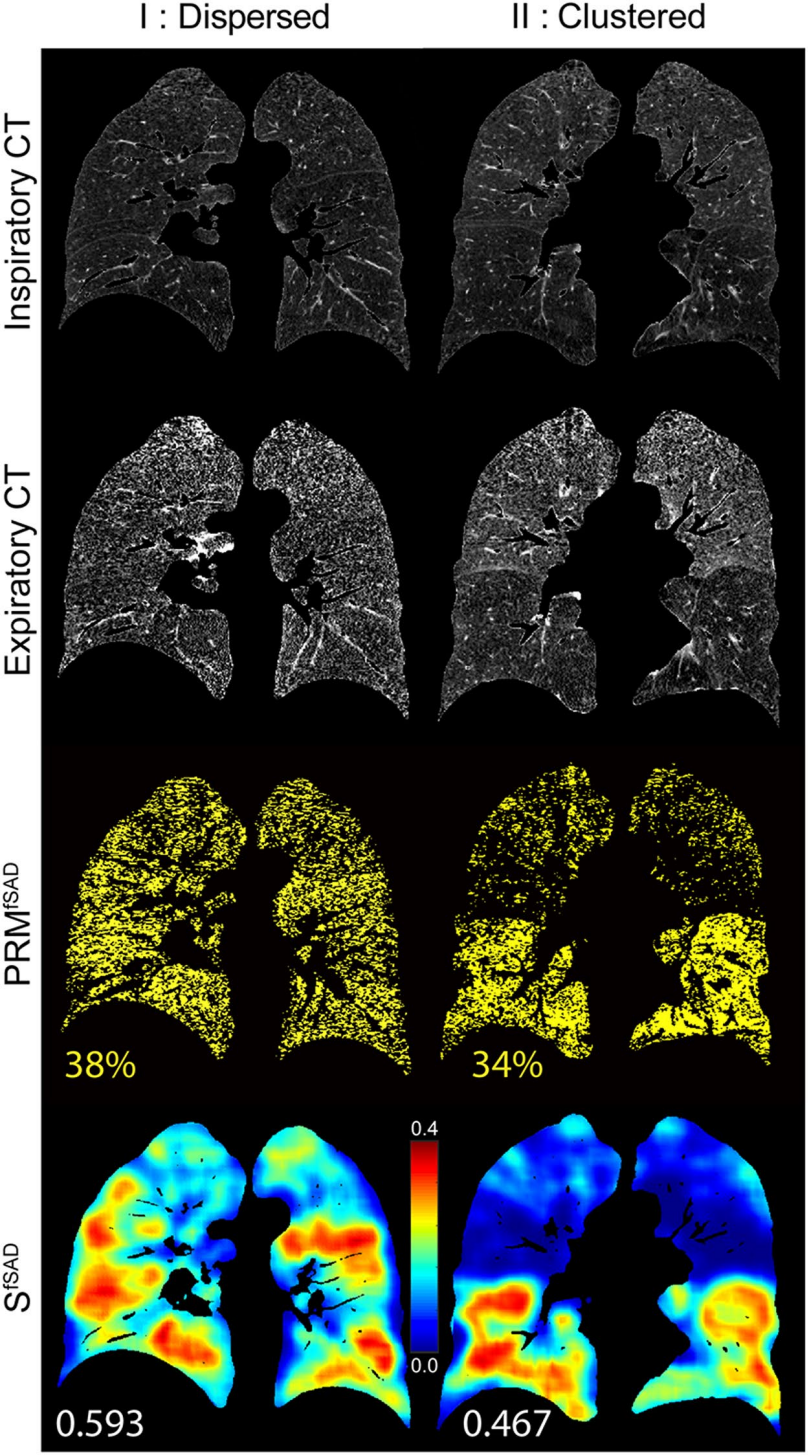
Two cases are presented with varying topological PRM features. Both cases have near-identical spirometry readouts and PRM^fSAD^ relative volumes yet display differing fSAD topological features: dispersed disease (I, left column) and clustered disease (II, right column). A representative slice is shown for each case for the respective (top to bottom) inspiratory CT, expiratory CT, PRM^fSAD^ map, and Local S^fSAD^ map (multiplied by Local V^fSAD^ in order to emphasize regions of substantial disease). PRM^fSAD^ relative volumes and Local S^fSAD^ values are displayed for comparison.
